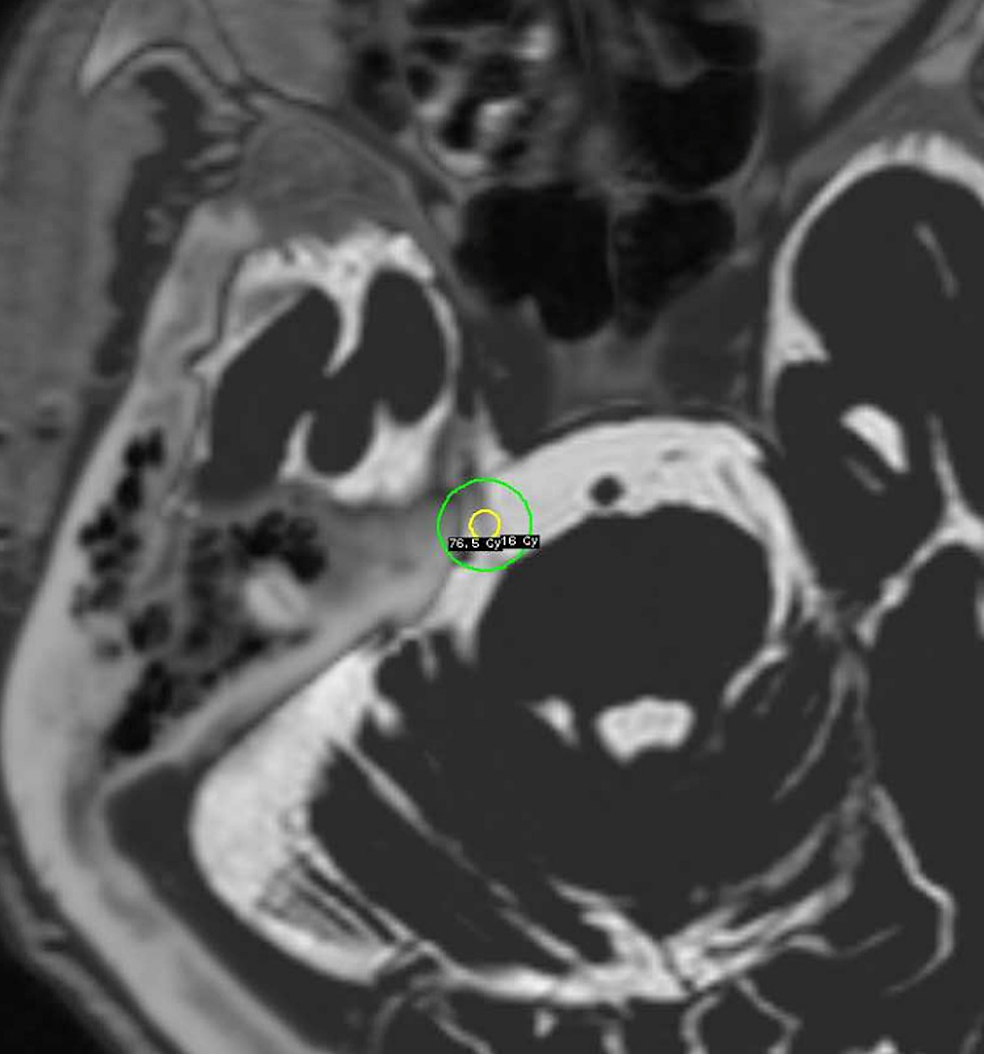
Dose plan of gamma knife radiosurgery by three-dimensional CISS images localized on MRI and CT under stereotactic conditions for trigeminal neuralgia CISS = Interference in steady state; MRI = Magnetic resonance imaging; CT = Computed tomography The cisternal portion of the trigeminal nerve, close to the Gasserian ganglion, was irradiated by a maximum dose of 85 Gy, using a single 4 mm collimator (yellow circle showing 90% isodose line and green circle showing 16 Gy isodose).
